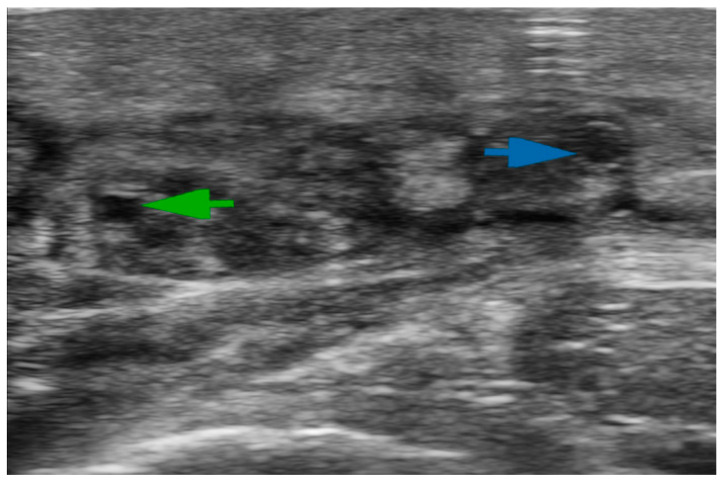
Ultra-high-frequency ultrasound (48 MHz probe) accurately reveals lymphatic vessels and neighboring veins. The green arrow indicates the lymphatic collector; the blue arrow shows the vein.
